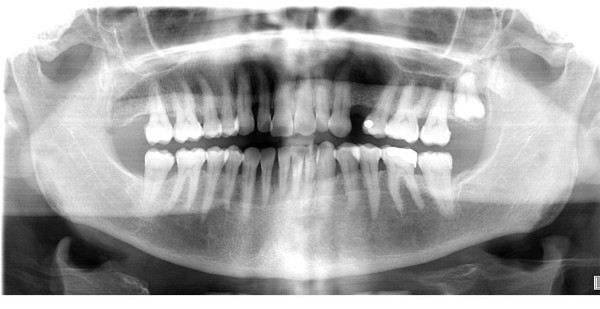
Panoramic radiography showing generalized alveolysis.
